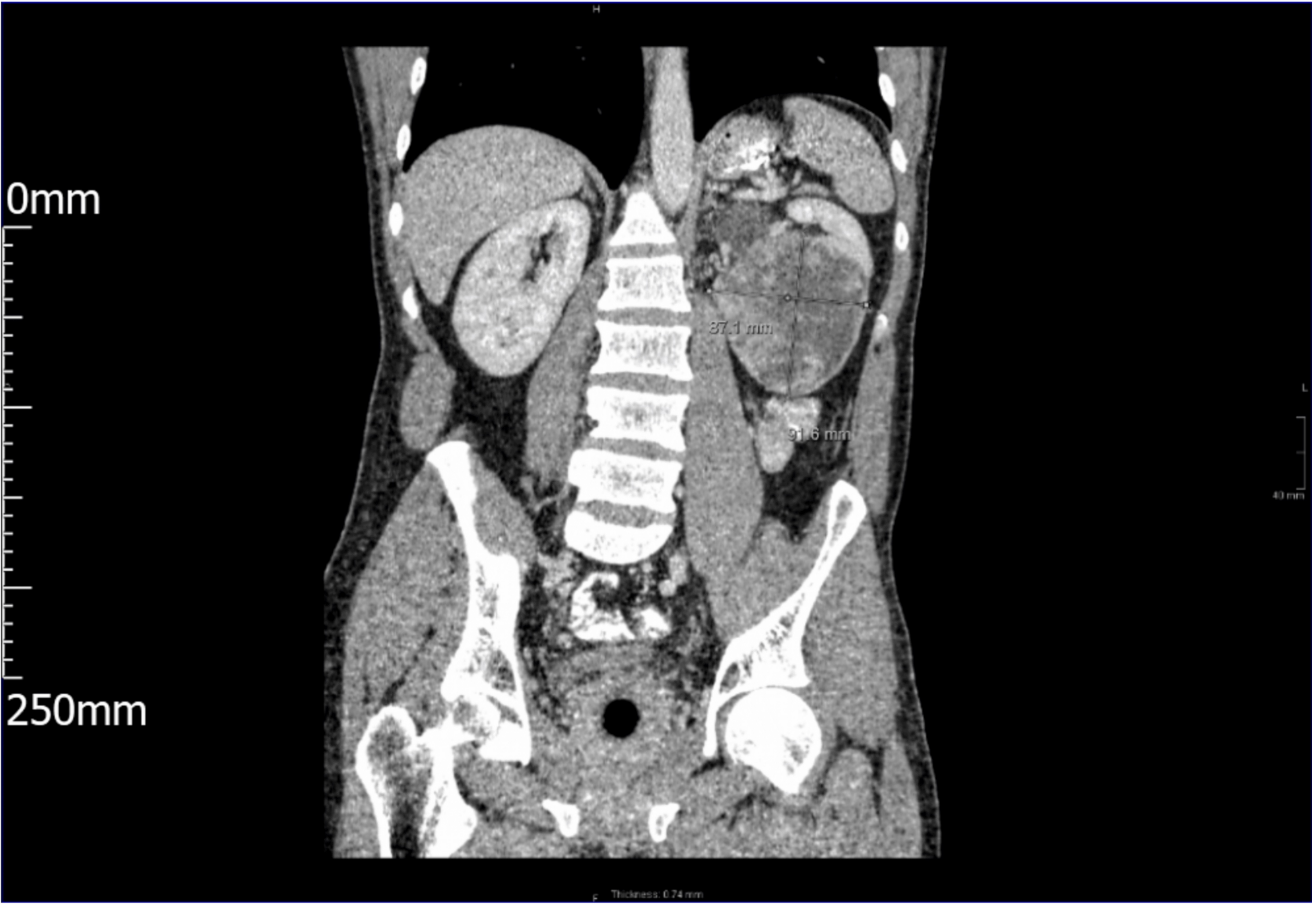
Longitudinal cross-sectional image from CT colonography.
